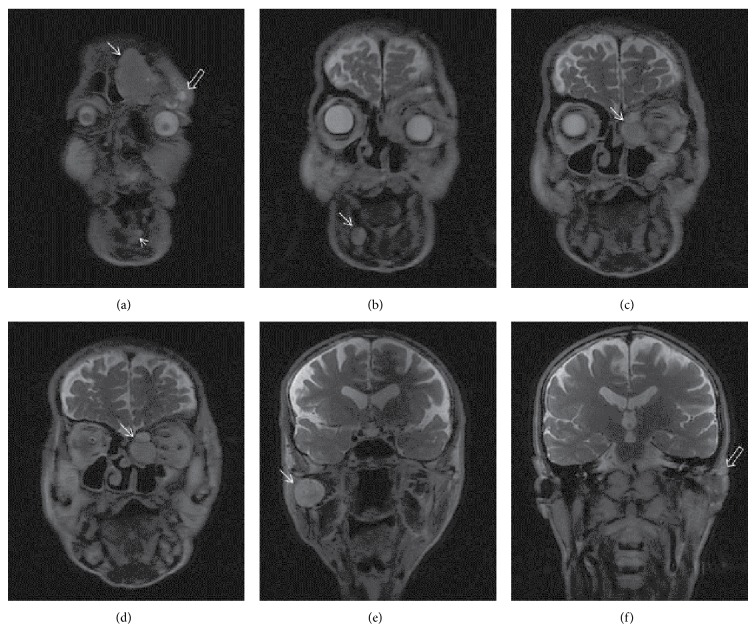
Coronal T2-weighed MR imaging: (a) a lobular hypodense mass caused destruction and expansion at frontal sinus like a mucosel or keratocyst (arrow mark). Cystic mass at left orbital wall and mandibular corpus (arrow marked). (b) Dens odontogenic keratocysts at right mandibular corpus (arrow mark). (c), (d) Multiple cystic lesions with different size and dens at left ethmoidal sinuses (marked arrow). (e) A round, dense cystic lesion with expansil regular contour was observed in the right mandibular ramus. (f) A soft tissue mass with heterogeneous and irregular contour, which contained cystic-necrotic areas starting from left auricula, extended to external ear tract (arrow mark).
